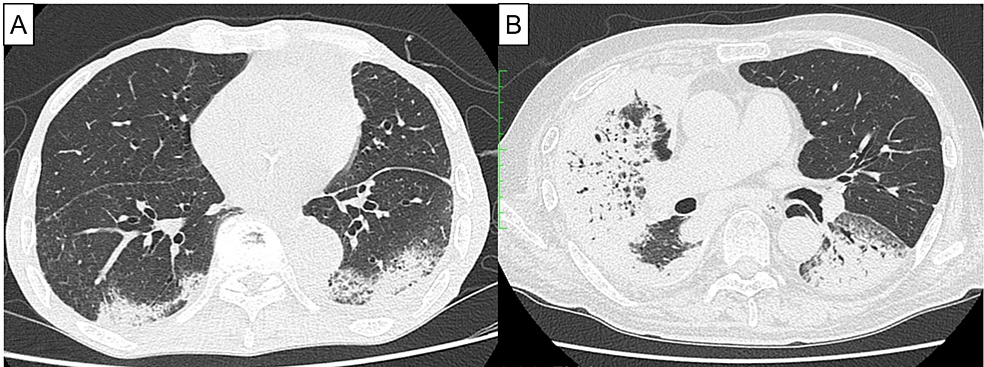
Chest CT at the onset of pneumonitis in two cases (A) A chest CT at the onset of pneumonitis in Case 1; (B) A chest CT at the onset of pneumonitis in Case 3
